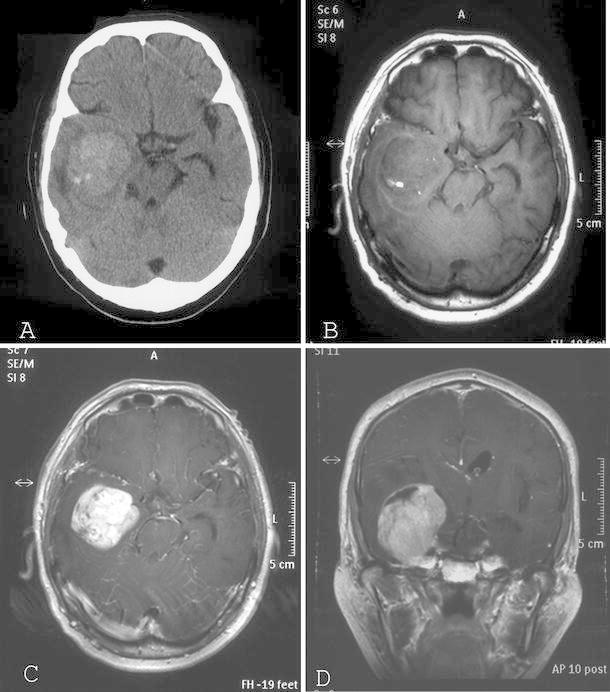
Preoperative imaging studies. a Axial-plane CT scan showing a high-density lesion in the right temporal lobe. b Axial T1-weighted MR image demonstrating the tumor with heterogeneous intensity located intraaxially and in the extraventricular space. c Axial contrast-enhanced T1-weighted MR image demonstrating a strongly enhancing mass. d Coronal contrast-enhanced T1-weighted MR image demonstrating a strongly enhancing mass. The tumor occupies the right temporal lobe
